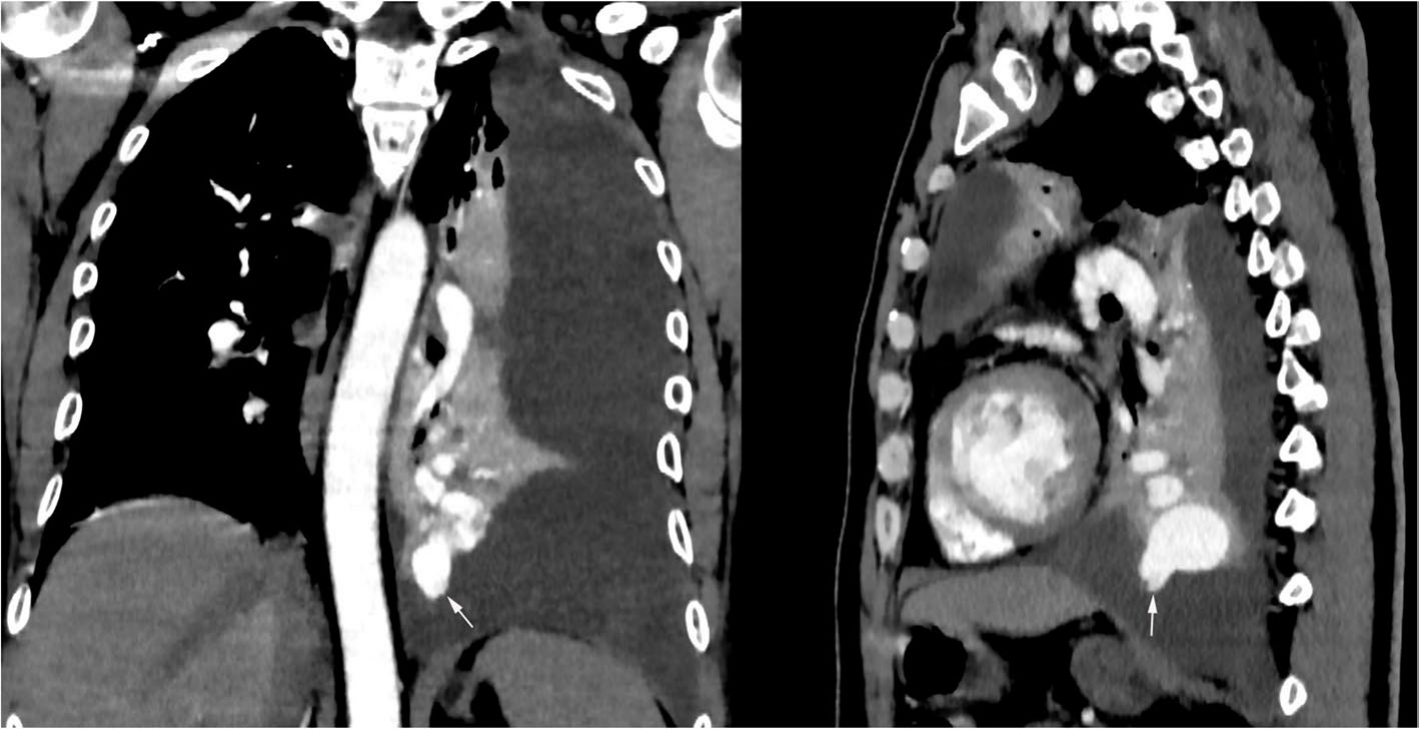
A-B Pulmonary arterial CT angiography demonstrated an irregularly enhanced mass communicating with the tortuous pulmonary vessels in the left lower lobe
